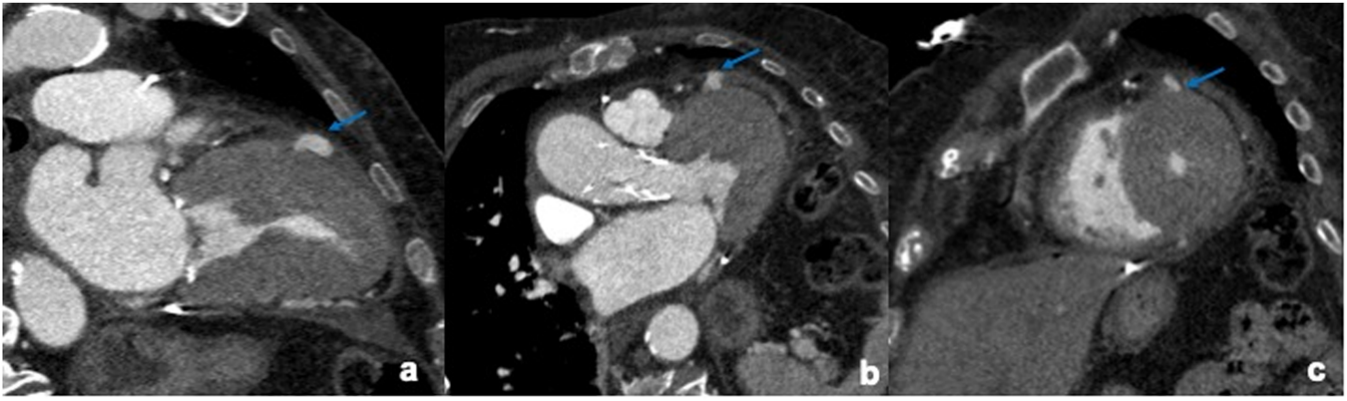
A 55-year-old female patient undergoing post-TAVI CTA for anemia onset (Hb: 9.5 g/dL) after the procedure showing focal blood leakage anteriorly to the left ventricle (a, b, c, arrow) due to an iatrogenic ventricular wall lesion
